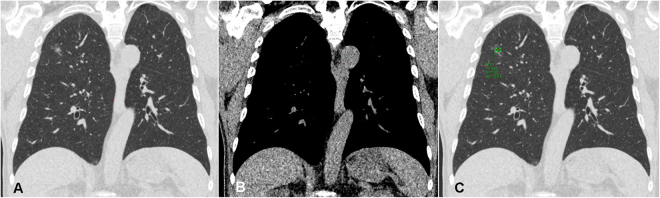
An example of heterogeneous GGN according to the SSN subclassification. A 66-year-old man had a 1.2 cm heterogeneous GGN nodule in RUL. The LDCT images showed heterogeneous groundglass opacities with focal solid component only when viewed on the lung window (Fig. 4A), but not seen on the mediastinal window (Fig. 4B). The average CT attenuation values (min, max and mean) expressed in HU were measured by placing an ROI of 15 mm^2^ on the lesion (Fig. 4C). In addition, to avoid placing the ROI box in or near the blood vessels could reduce the measurement errors. The patient underwent video-thoracoscopic wedge resection of RUL. Further pathologic report demonstrated invasive pulmonary adenocarcinoma in RUL. Abbreviations: GGN: groundglass nodule; SSN = subsolid nodule; RUL = right upper lobe; ROI = region of interest; HU = Hounsfield unit.
